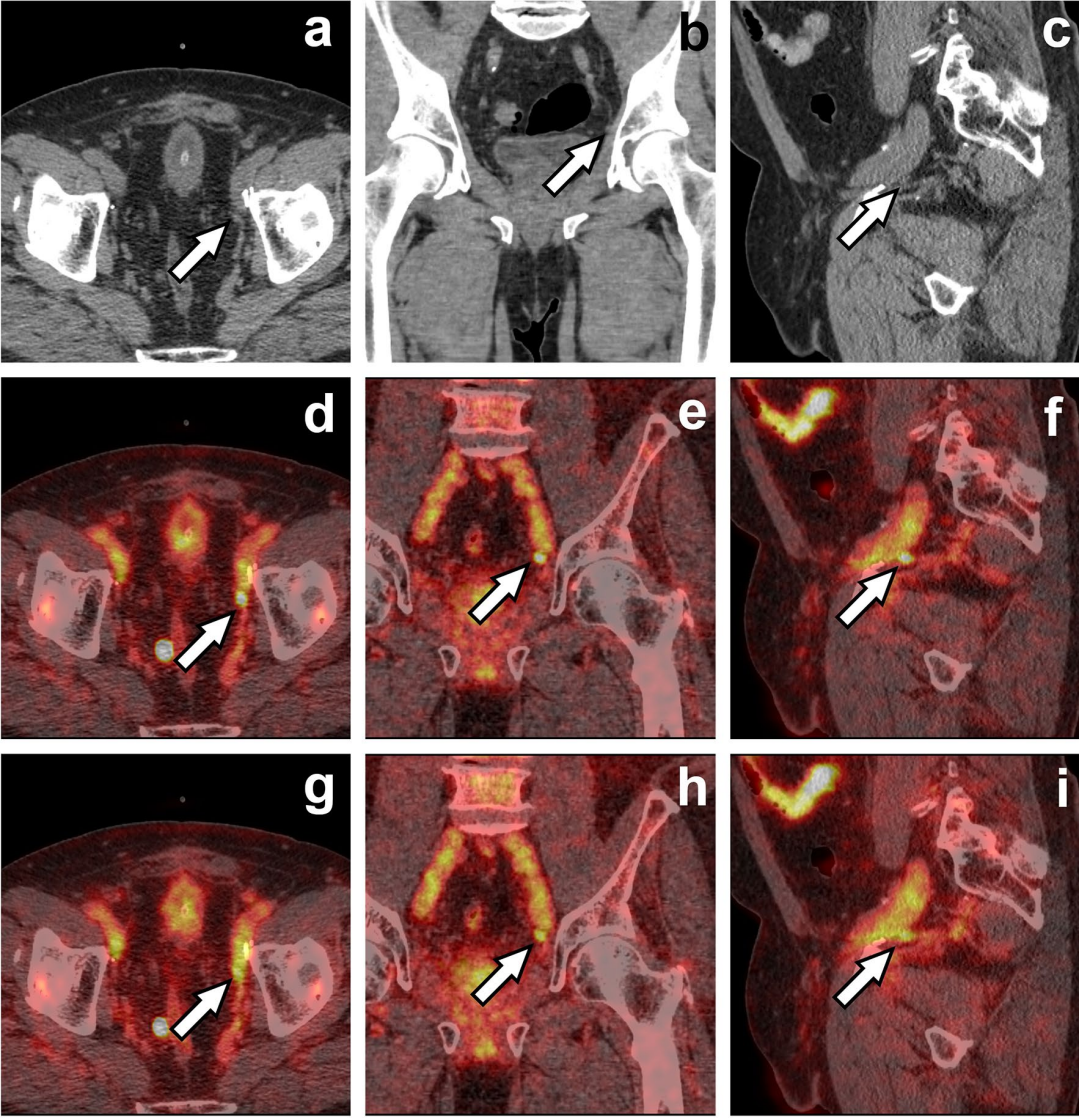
A 60-year-old prostate cancer patient after radical prostatectomy and pelvic radiation beam therapy with biochemical relapse (PSA 0.72 ng/ml). Axial, coronal and sagittal CT (a–c), Q.Clear fused PET/CT (d–f) and OSEM fused PET/CT (g–i) images are presented. White arrow indicates a tracer-avid left internal iliac node (6 mm in short axis) which seems more distinct on Q.Clear images (d–f). The node also had higher Q.Clear than OSEM values of SUVmax (5.3 vs 4.9), SUVmean (3.3 vs 2.8) and TBR (25.0 vs 17.7)
